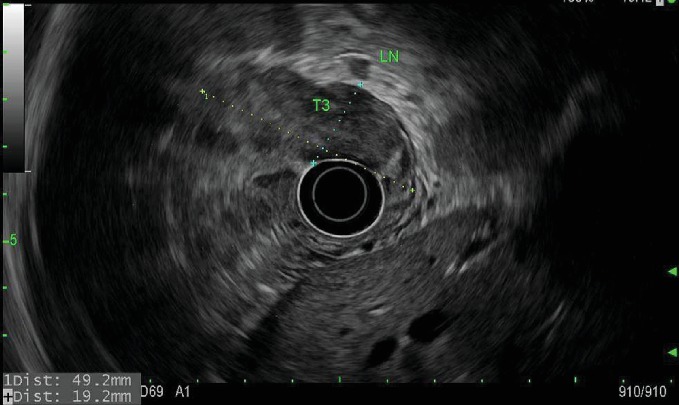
EUS—a hypoechoic mass involving two-thirds of the gastric circumference measured 4.8 × 1.9 cm, with serosal invasion and two malignant-appearing lymph nodes (one shown in field-of-view) in the paracardial region (level 16), T3N1MX by endosonographic criteria.
